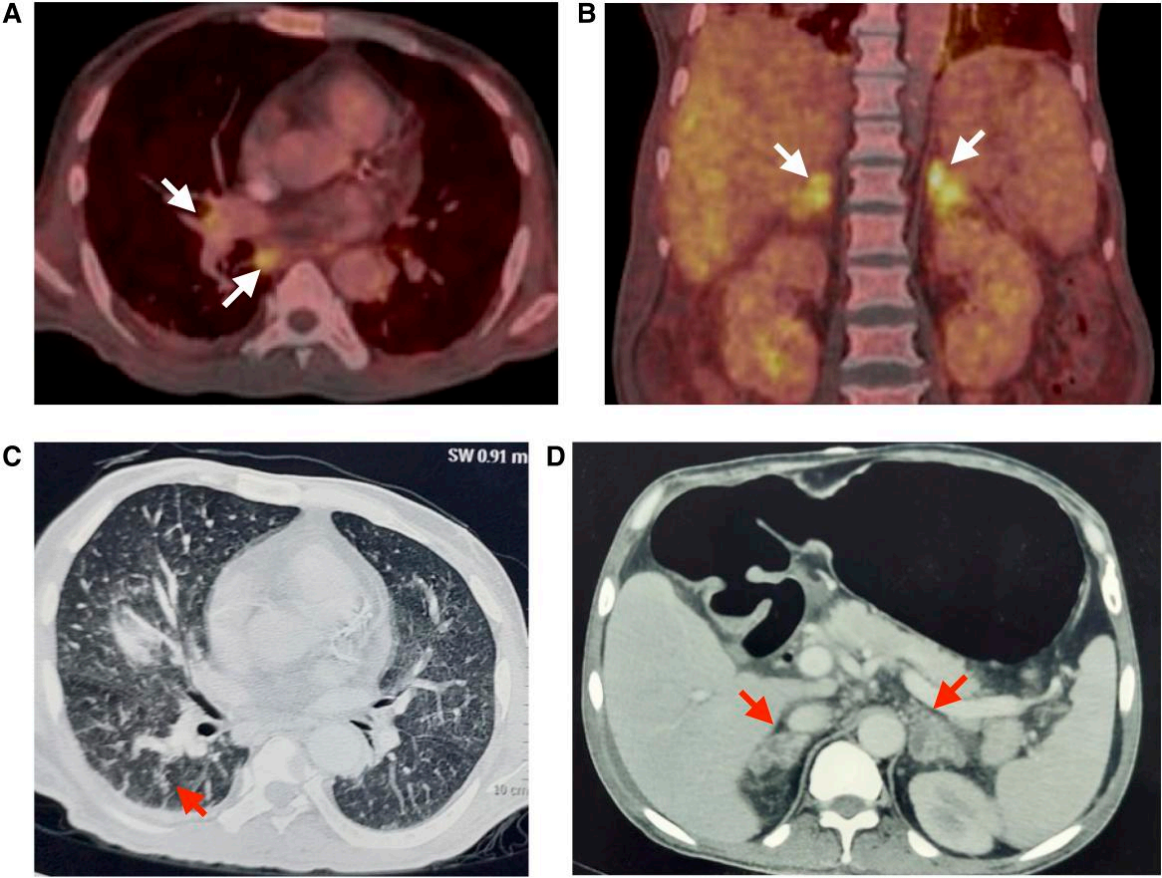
Radiological evaluation of the patient. A, Axial section of ^18^F-FDG/PET-CT scan showing FDG-avid hilar and subcarinal lymph nodes (arrows). B, Coronal section of ^18^F-FDG/PET-CT scan showing diffuse symmetric FDG avidity in bilateral adrenal glands which are enlarged (arrows) and hepatosplenomegaly. C, Axial section of CECT scan showing centrilobular nodules in right lung lower lobe (arrow). D, Axial section of CECT scan showing both adrenal glands that are enlarged with internal areas of necrosis (arrows). Abbreviations: ^18^F-FDG/PET-CT, ^18^F-fluorodeoxyglucose positron emission tomography-computed tomography; CECT, contrast-enhanced computed tomography.
